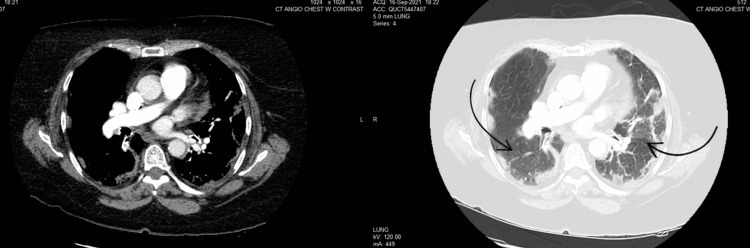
CT angiogram: bilateral multifocal pneumonia (black arrows) with no evidence of acute pulmonary embolism.
